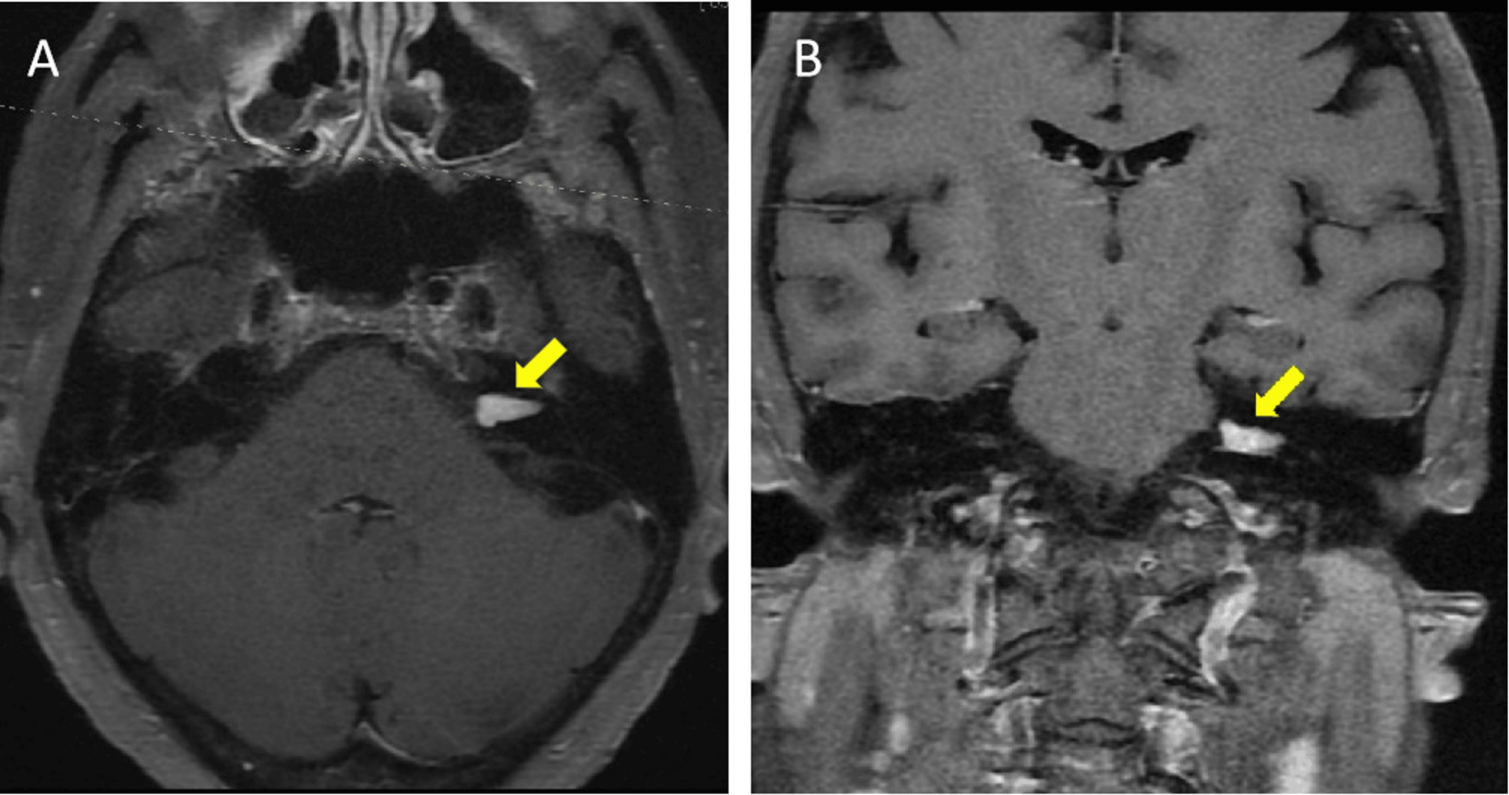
MRI brain T1 post-contrast series A 12 × 5 × 5 mm avidly enhancing mass filling the left internal auditory canal, extending slightly beyond the porus acusticus into the cerebello-pontine angle cistern. (A) Axial view. (B) Coronal view.
